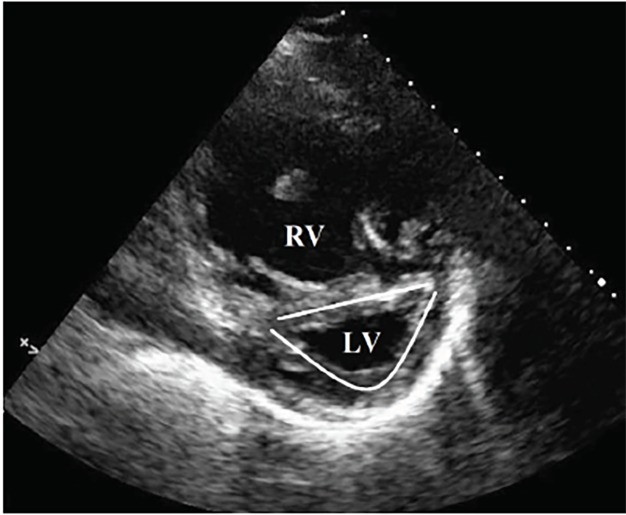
Transthoracic echocardiogram parasternal short view demonstrating enlargement of the right ventricle (RV) with bowing of the interventricular septum (straight line) toward the left ventricle (LV), demonstrating the “D” sign (outlined) of right ventricular overload.
